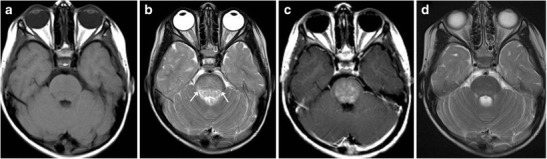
Isolated brainstem ADEM in a 12-year-old patient: a–c Axial T1-weighted image is unremarkable with hyperintense signal on T2 (*arrows*) involving the pons, which shows avid post-contrast enhancement. d Post treatment: Axial T2-weighted image shows near total resolution of changes in the pons
